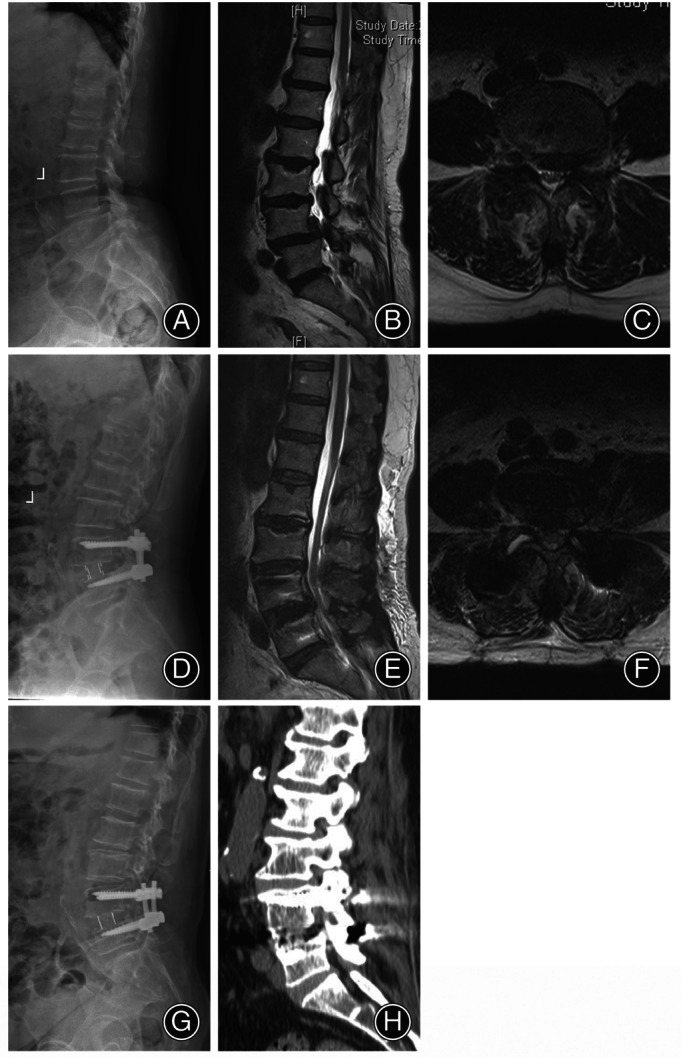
59‐year‐old female, chronic low back pain with left lower extremity radiating pain treated by ALDF. (A) Lateral radiograph shows grade II degenerative spondylolisthesis. (B, C) The sagittal and axial MRI images demonstrate central canal and left lateral recess stenosis with sequestrated disc at the L4/5 level. (D–F) X‐ray film shows excellent restoration of disc height and pedicle screw position, and MRI images show complete decompression and recovery of the cross‐sectional area 6 months after surgery. (G, H) Three‐year postoperative X‐ray and CT images show the achievement of interbody fusion.
